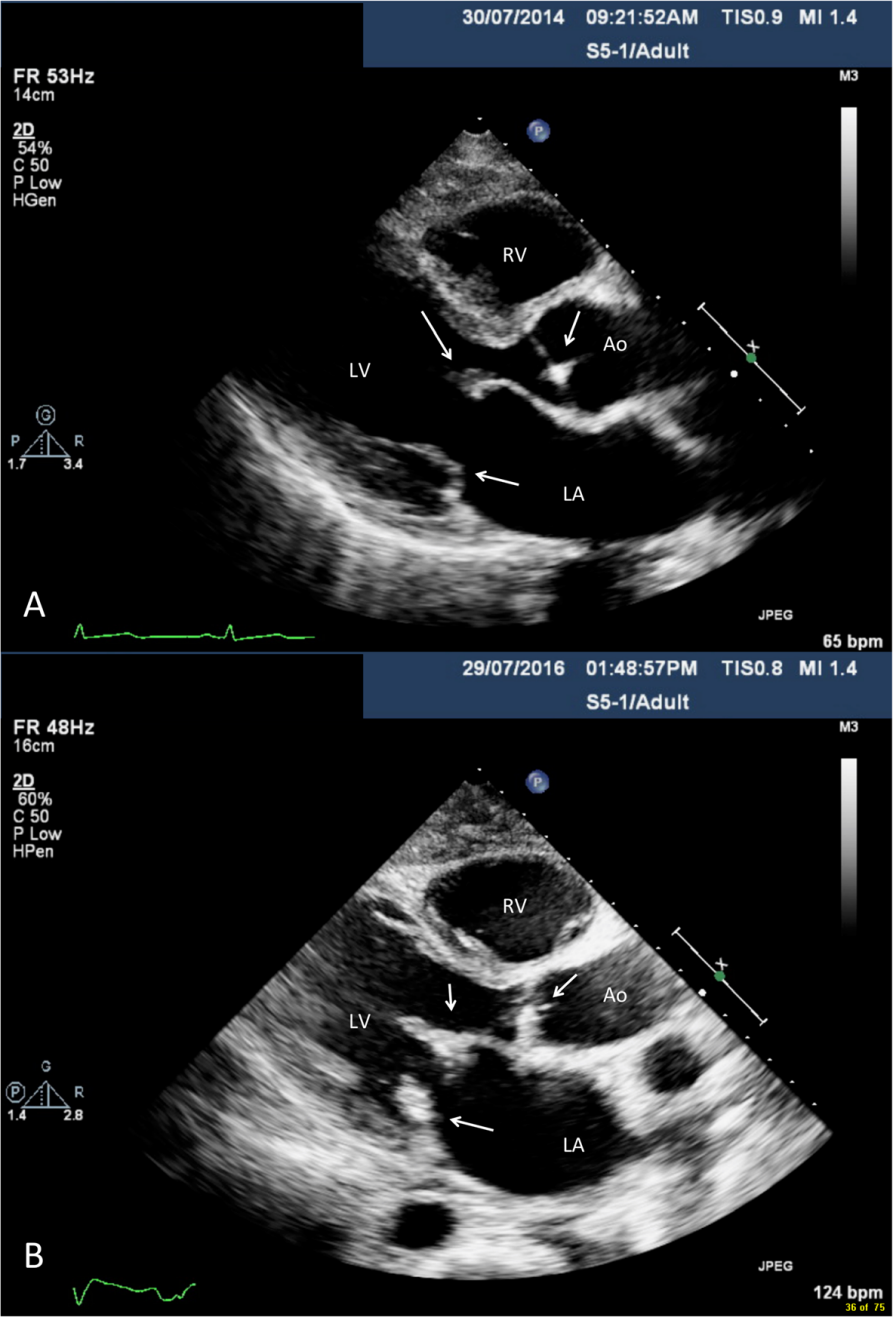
Parasternal long axis transthoracic echocardiogram findings in 2014 (a) and 2016 (b). Thickened mitral and aortic valve leaflets with diastolic doming of the mitral valve is depicted. Notably, in 2016 there was substantial interval thickening of the mitral and aortic valve leaflets
